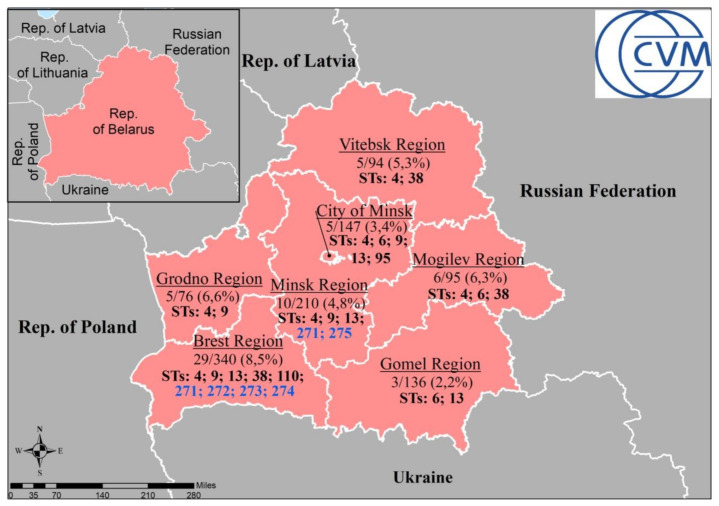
Geographical distributions of *C. trachomatis* STs in the Republic of Belarus among CT-positive patients/totally examined (%). The map was generated with Esri ArcGis Desktop 10.6.1 (www.esri.com (accessed on 10 February 2022)). Novel STs identified in this study are marked in blue.
